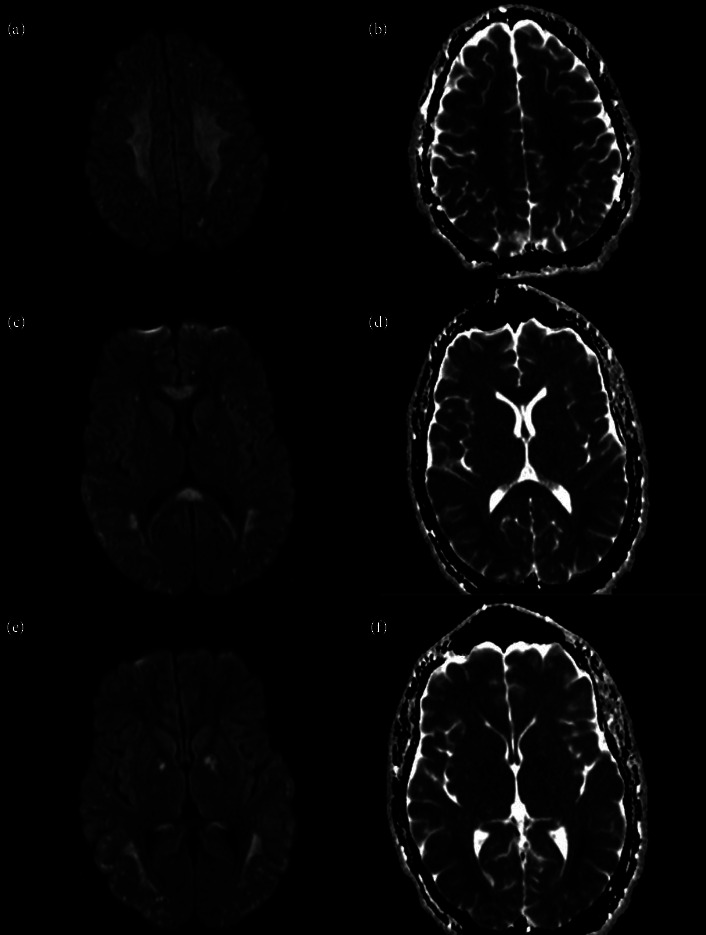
Magnetic resonance imaging (MRI) of the brain showing diffusion weighted imaging (DWI; images (a), (c), and (e)) and apparent diffusion coefficient (ADC; images (b), (d), and (f)) sequences. Restricted diffusion noted in the subcortical white matter (a and b), corpus callosum (c and d), and globus pallidus (e and f).
